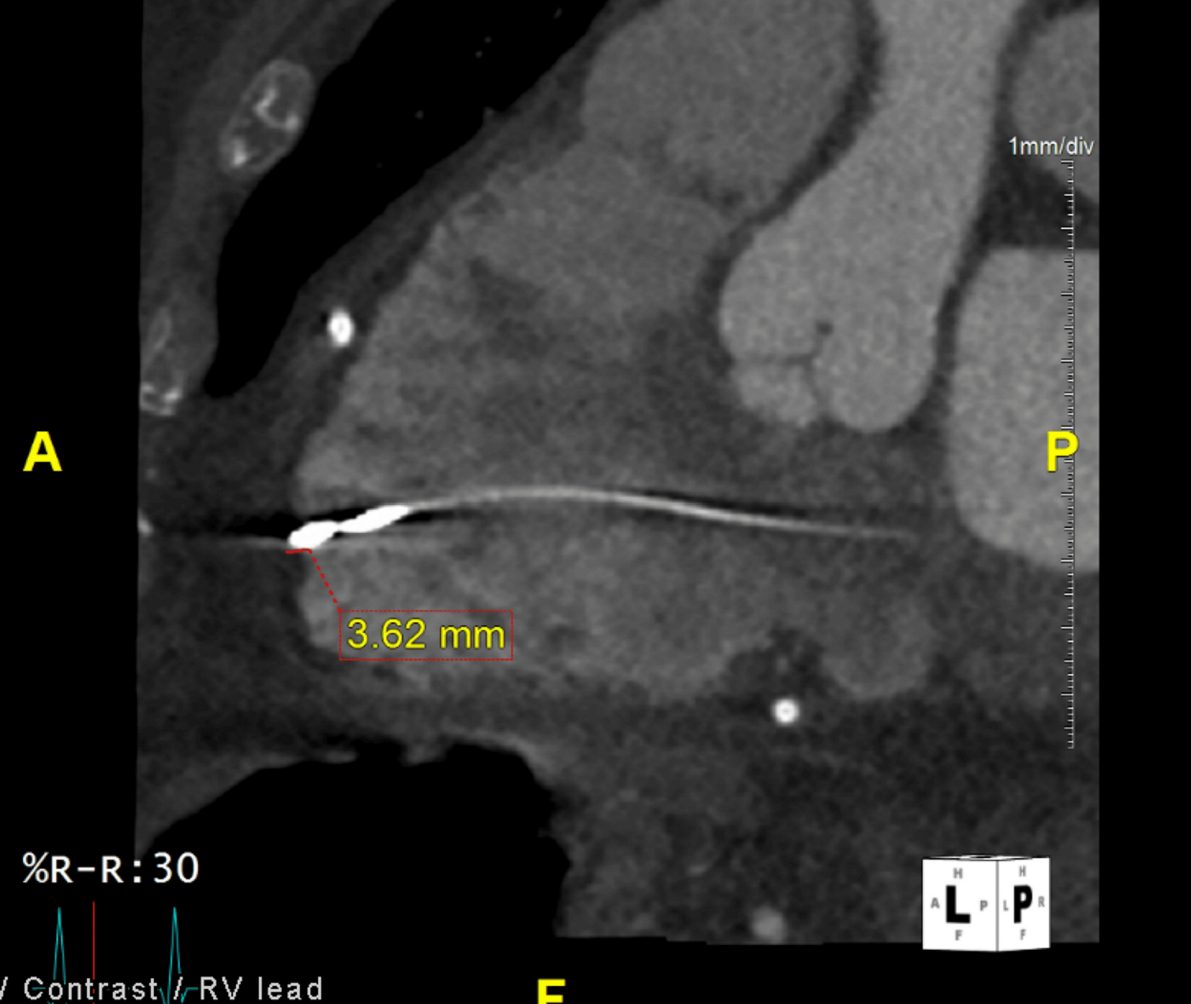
Computed tomography scan depicting right ventricular (RV) wire extending 3.62 mm through the wall of the RV.
